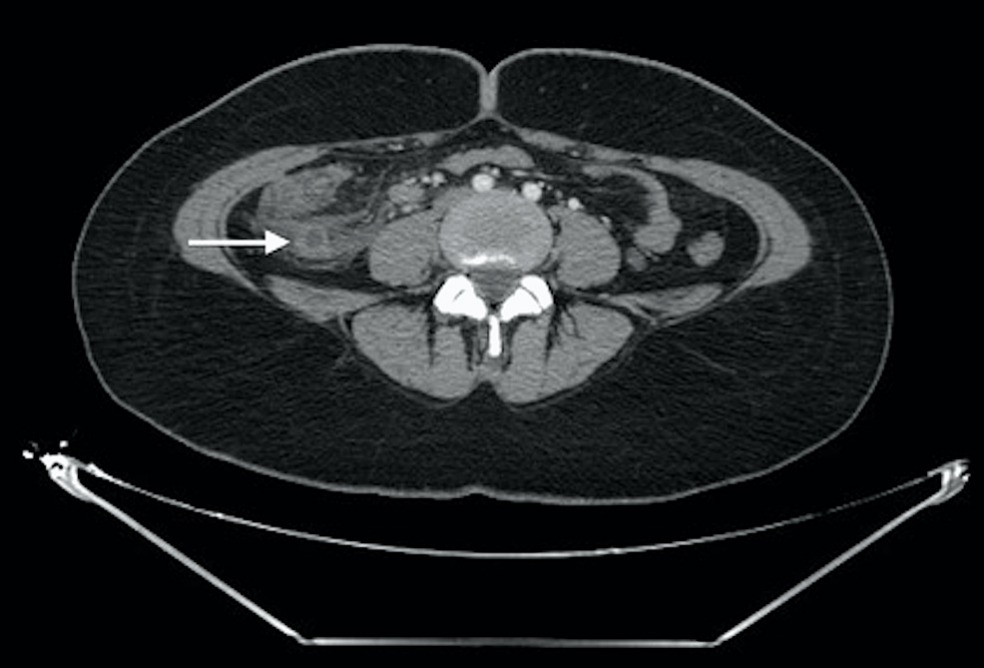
Axial CT scan showing swollen appendix surrounded by mesenteric fat infiltration and peritoneal effusion: Acute appendicitis (white arrow) CT: Computed tomography
